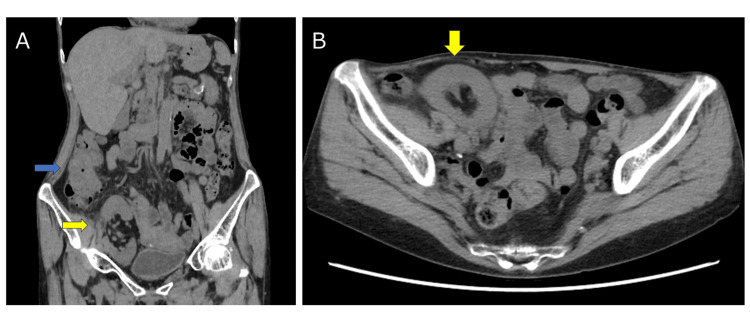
Computed tomography (CT) findings Non-contrast CT showed an ascending colon tumor (blue arrow) and a renal allograft in the right iliac fossa (yellow arrows). (A) Coronal view; (B) Axial view
